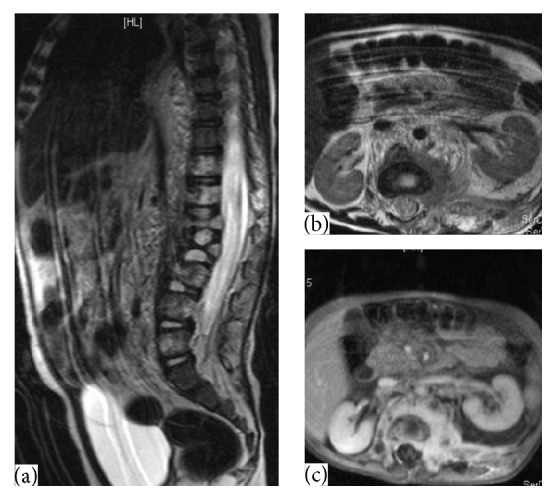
Partial response after interferon-*α* therapy. Sagittal and transverse MR images show high signal intensity on T2-weighted image (a-b). Transverse contrast-enhanced T1-weighted image shows homogeneous enhancement of the lesions (c).
